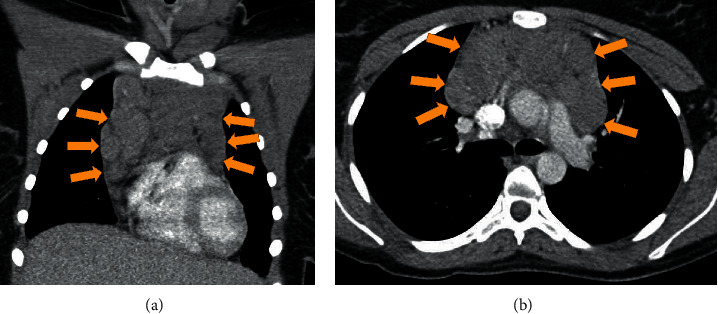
CT thorax demonstrating multiple pathologically enlarged left supraclavicular lymph nodes associated with enlarged partially visualized left cervical lymph nodes. Mass effect on the left lobe of the thyroid gland and the trachea from the pathologically enlarged left supraclavicular lymph nodes. Pathologically enlarged lymph nodes throughout the anterior mediastinum. Enlarged right paratracheal lymph nodes as well. Pathologically enlarged left axillary and subpectoral lymph nodes as well.
